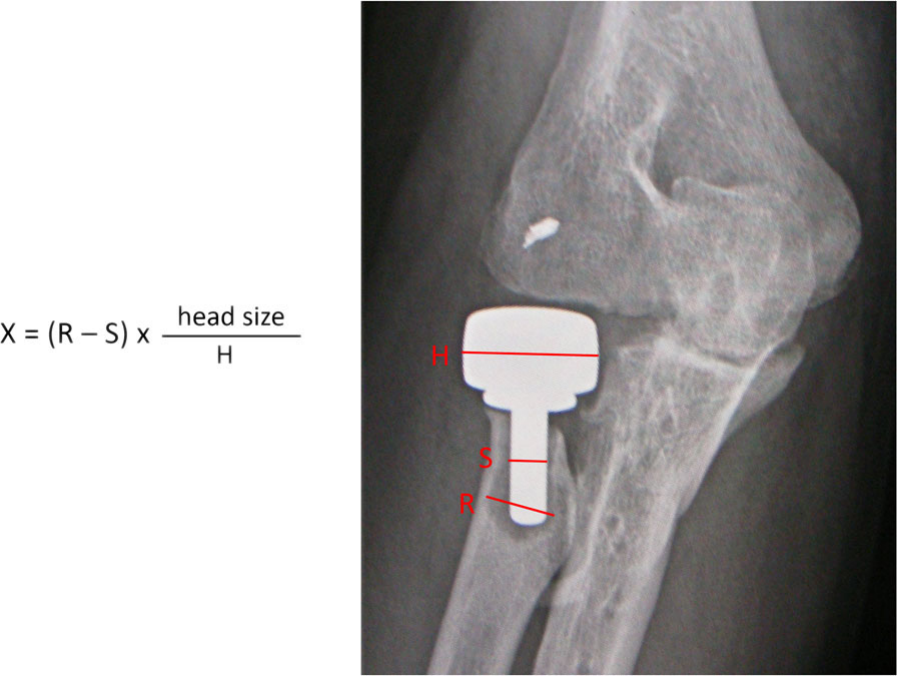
Thickness of radiolucency (*X*) was calculated using the elbow radiograph. R, maximal width of decreased density around the stem. S, width of the stem. H, width of the head. Head size was the diameter of radial head prosthesis according to the surgical record (unit, mm)
